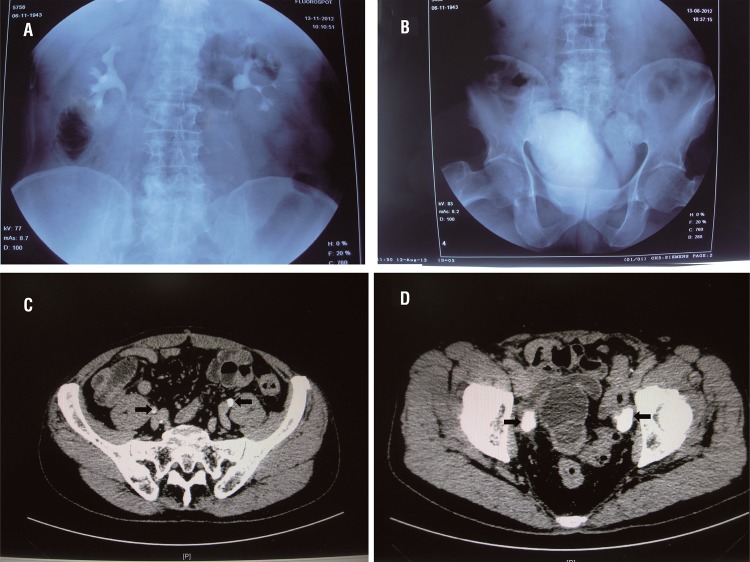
Image examinations from follow-up. A) An IVP showed the bilateral pelvis and ureters were normal and there were no appearance of hydronephrosis (POD 10 months). B) An IVP showed the shape of the neobladder. C) Delay phase of a enhanced CT scan showed there were no appearance of the dilation of the bilateral ureters (black arrows). D) Delay phase of the enhanced CT scan showed the appearance of the bilateral isoperistaltic afferent limbs (black arrows) and the neobladder.
